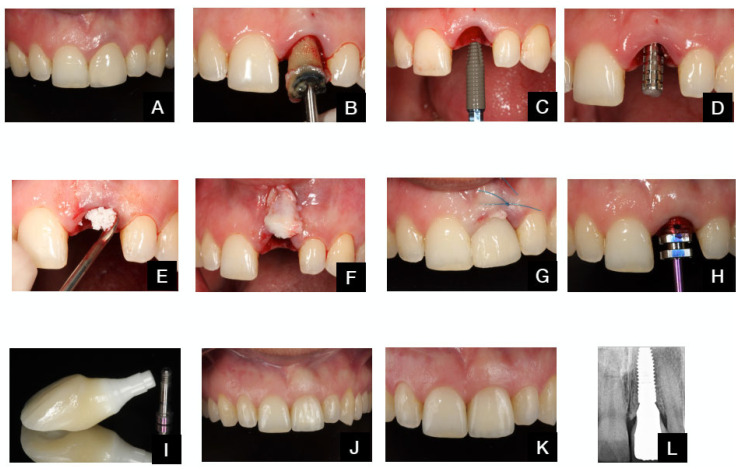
TAOM protocol. (A). Pre-op situation. (B). Tooth extraction. (C). Immediate implant placement. (D). Provisional abutment. (E). Bone graft in the del buccal gap. (F). Connective tissue-free palatal graft before insertion in tunnel preparation. (G). Grafts and provisional restoration in place. (H). Final impression. (I). Final restoration. (J). Final restoration delivered after 4 months. (K). Outcome after 12 months. (L). Final radiograph after 12 months.
